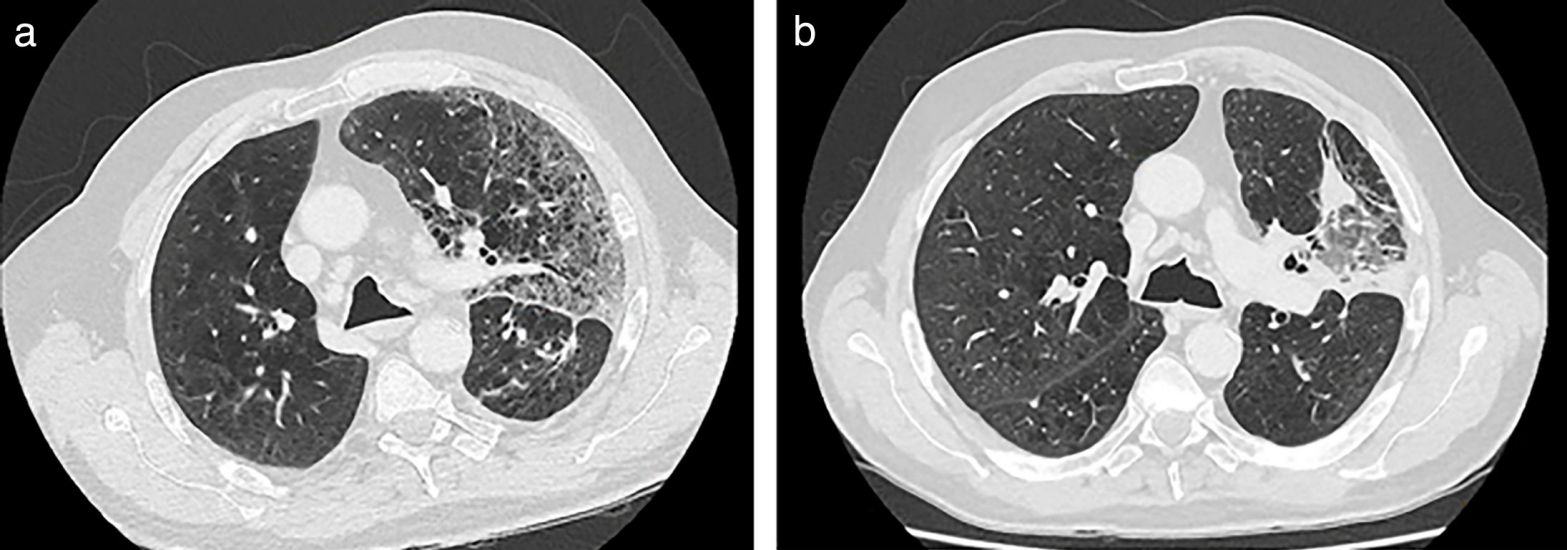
(a) Patient 1, CT chest pre-treatment. Diffuse ground-glass changes with interlobular septal thickening in left upper lobe, indicative of acute inflammation or infection. (b) Patient 1, CT chest post-treatment. Improvement in ground-glass opacities, traction bronchiectasis, and scarring in the posteroinferior aspect of the left upper lobe.
